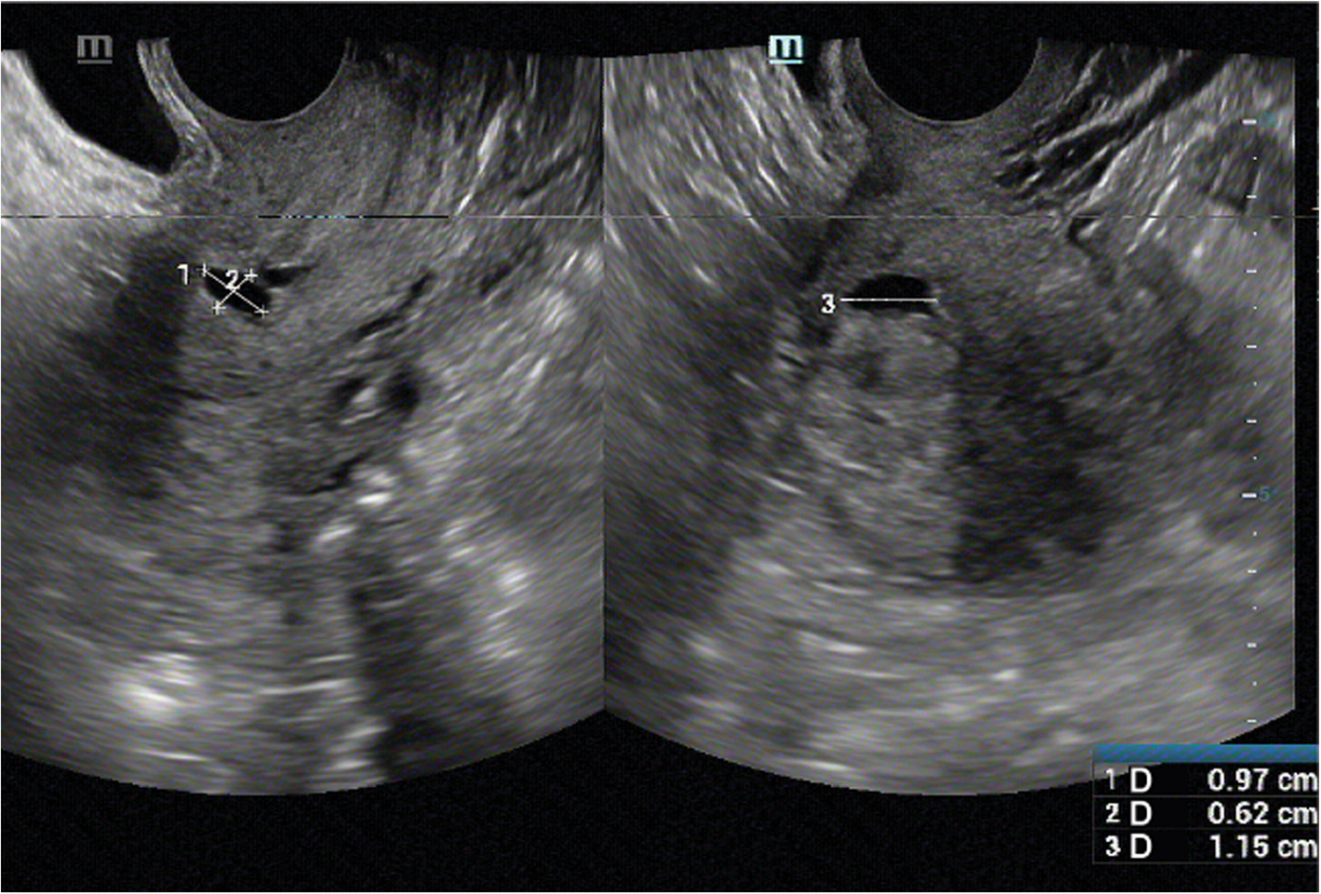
Ultrasound images of a cesarean scar defect. Size of scar defect (Length* Width* Depth):9.7* 11.5 *6.2 mm
